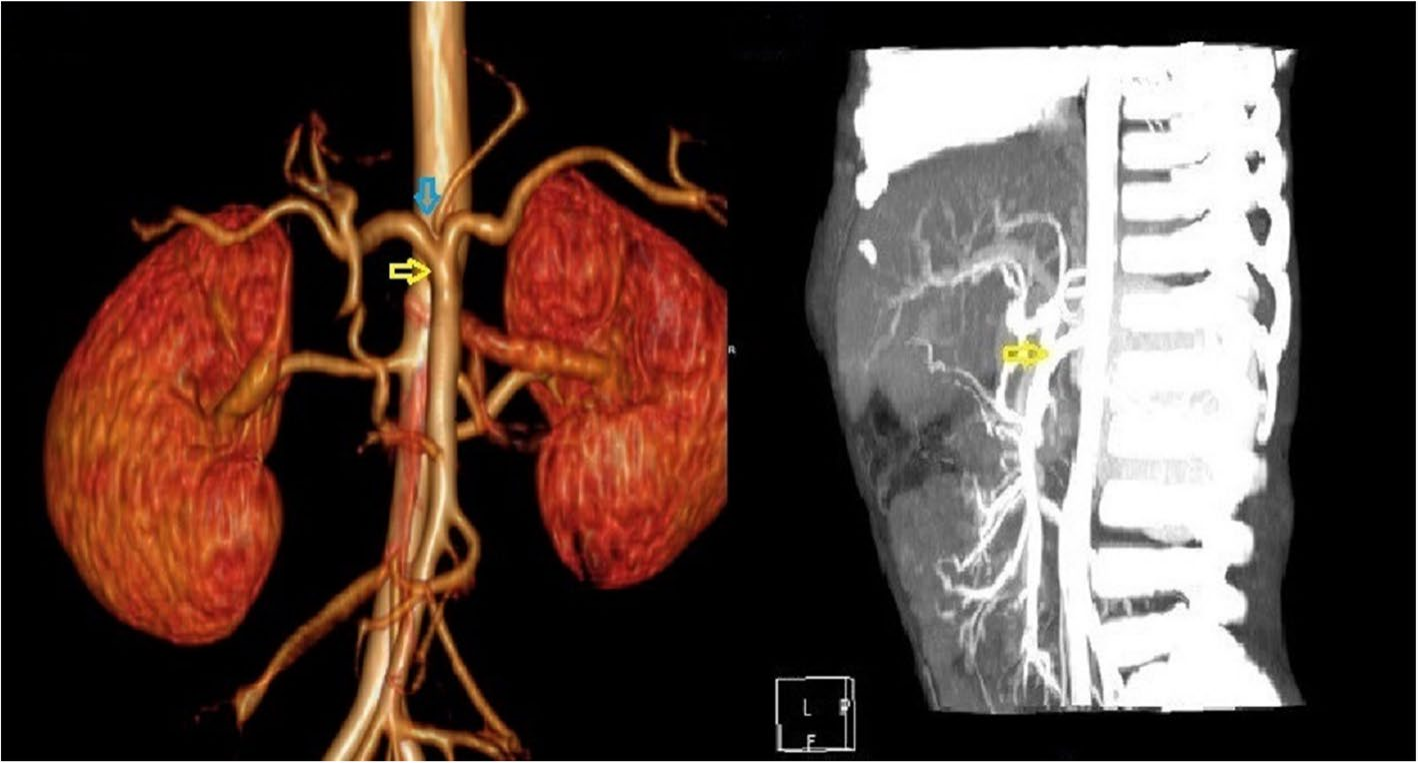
Abdominal computed tomography angiography shows that the celiac trunk and proximal superior mesenteric artery had a common trunk (CMT) (yellow arrow). The left gastric artery originated from the abdominal aorta (blue arrow)
